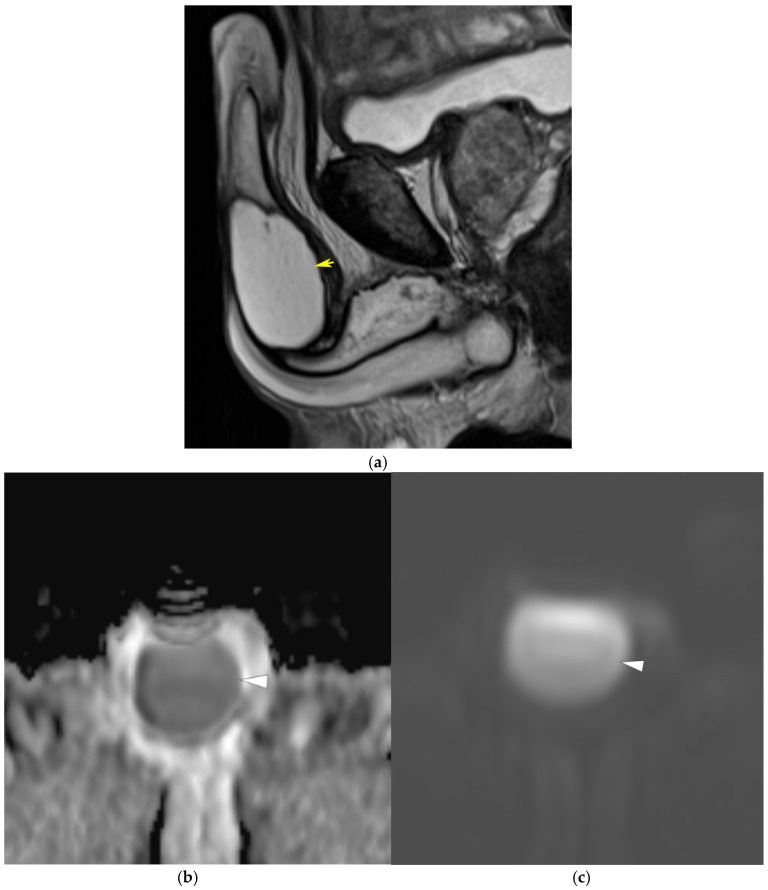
Penile abscess. (a) Sagittal T2-weighted image demonstrates a fluid-filled mass in the mid-portion of the penis (yellow arrow). (b) Corresponding apparent diffusion coefficient (ADC) map and (c) diffusion-weighted image reveal marked diffusion restriction within the lesion (white arrowhead), consistent with abscess formation.
